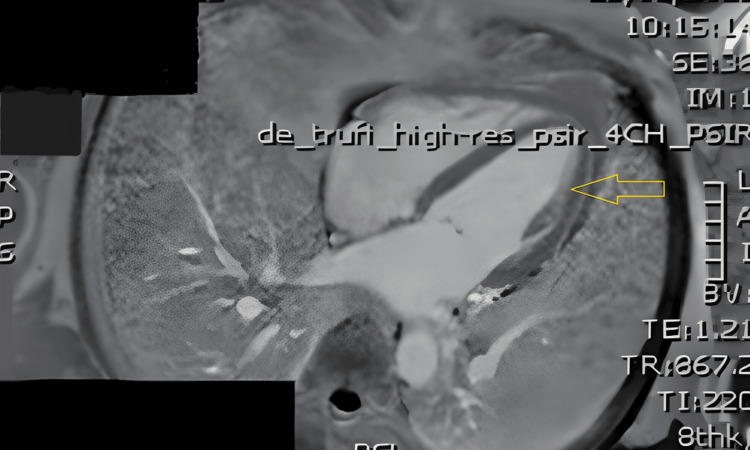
Cardiac MRI showing no evidence of myocardial scar or focal fibrosis. TrueFISP (TRUFT) cine cardiac MRI sequence demonstrating normal cardiac structure and function, with a normal-appearing left ventricular (LV) myocardium (yellow arrow).
